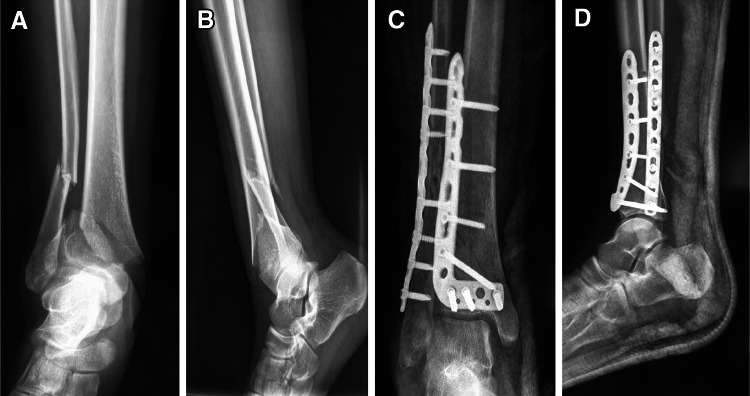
A 45-year-old man was involved in a car crash sustained a closed distal tibia-fibula fracture, the surgery was performed 10 days post-injury with the single anterior-lateral approach. a AP radiograph, b lateral radiograph, c after the fixation of plates via the single anterior-lateral incision, AP radiograph and d lateral radiograph
